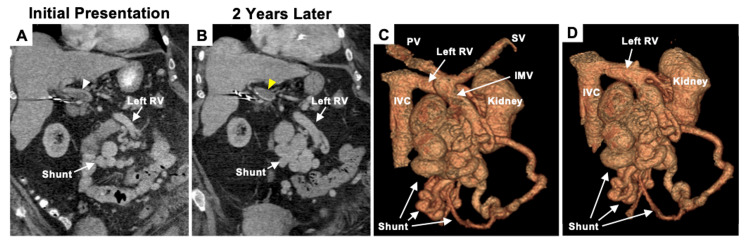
Chronic portal vein thrombosis and 3D reconstruction of the mesorenal shunt. Coronal CT of the abdomen/pelvis without contrast showing (A) nonocclusive portal vein thrombosis (white arrowhead), (B) completely occlusive portal vein thrombosis two years later, and a cross-section of the developing mesorenal shunt. (C) 3D reconstruction of the IMV-RV shunt. (D) 3D reconstruction of the IMV-RV shunt with the IMV, PV, and SV removed showing the shunt connecting with the left RV. CT: computed tomography; IVC: inferior vena cava; PV: portal vein; RV: renal vein, IMV: inferior mesenteric vein; SV: splenic vein
